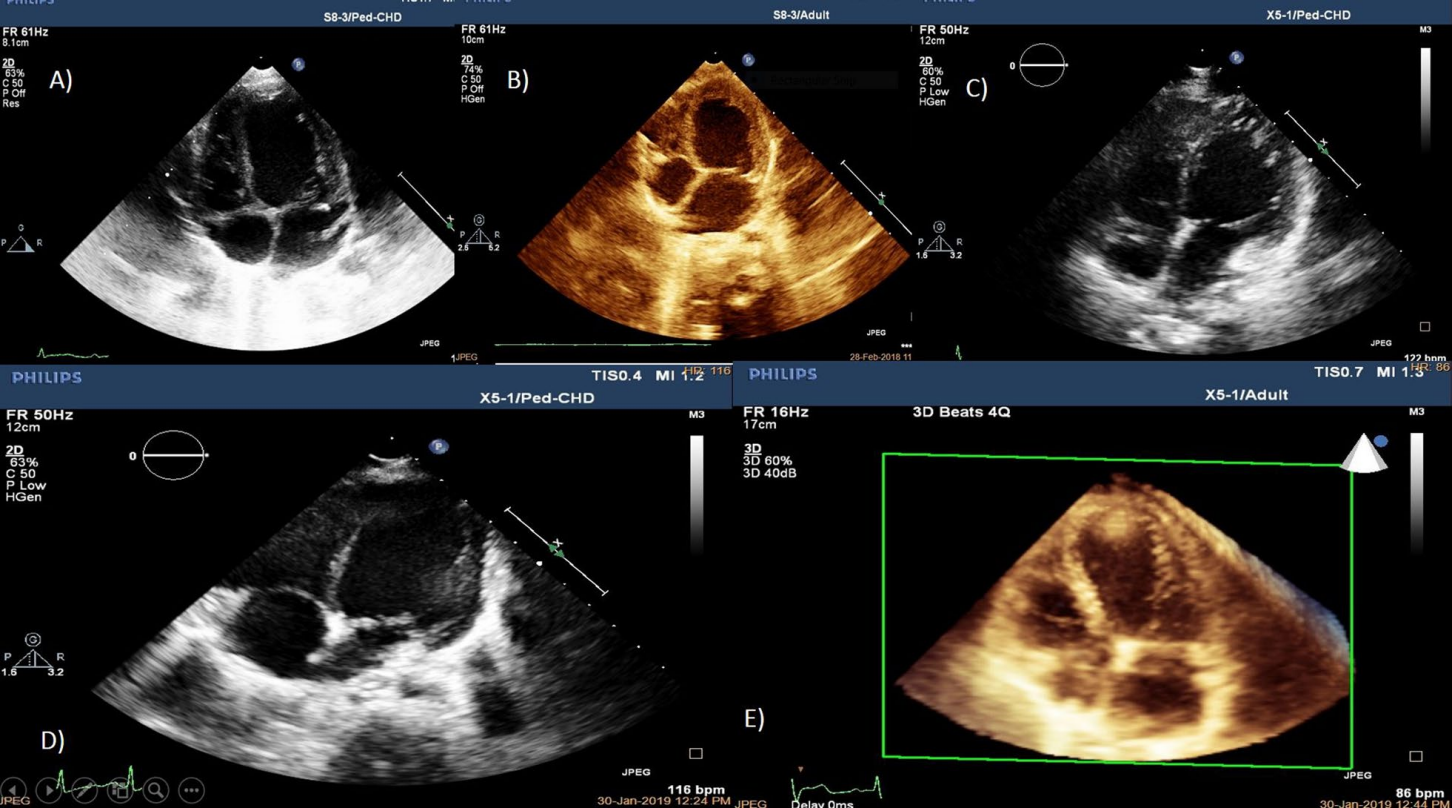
Left ventricular dilatation of patients with Alström syndrome on echocardiography. a Patient C, b Patient B, c Patient C, d Patient E, e Patient F
